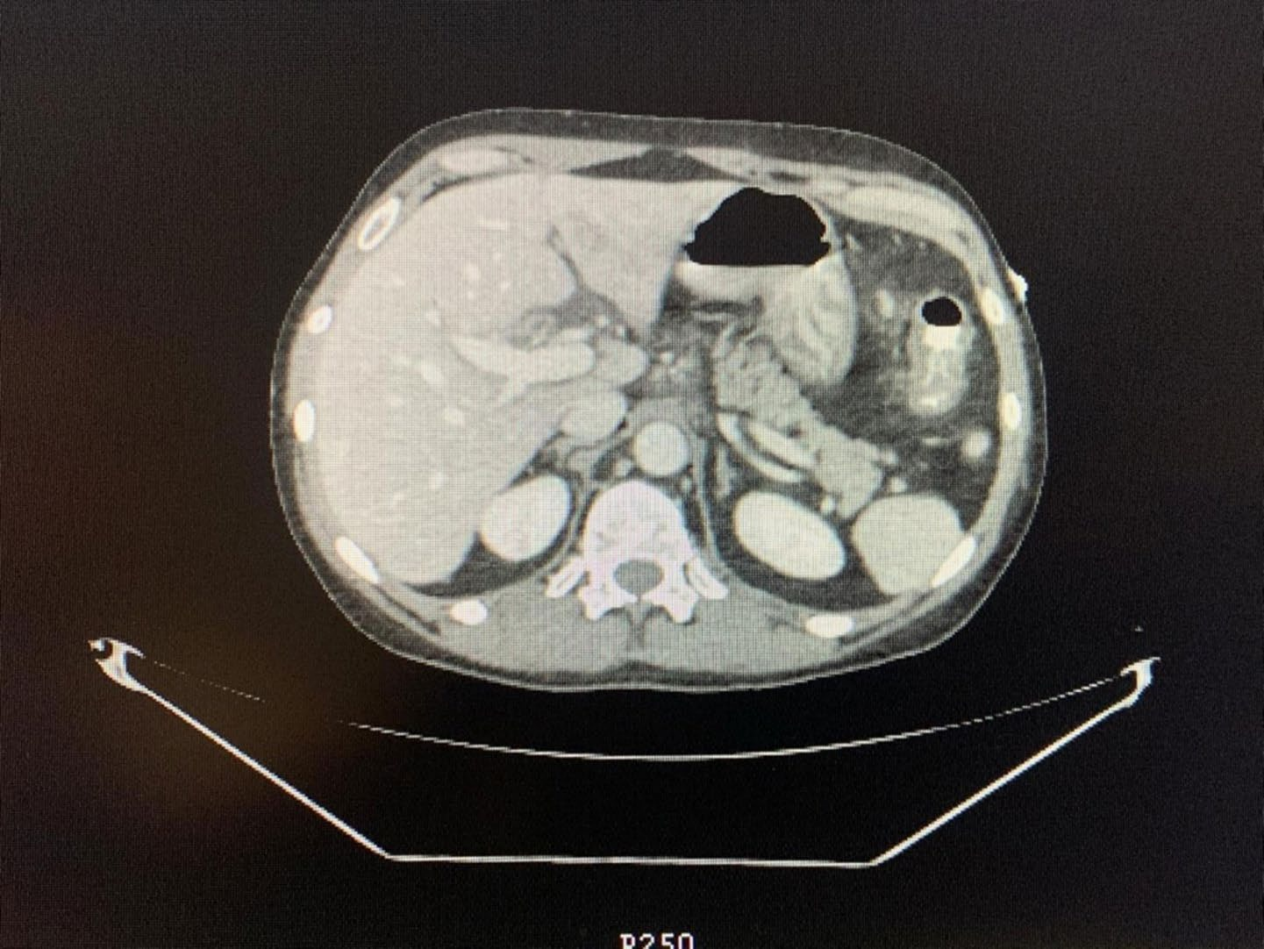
Abdominal CT scan: axial computed tomography (CT) image of the abdomen showing key anatomical structures.
